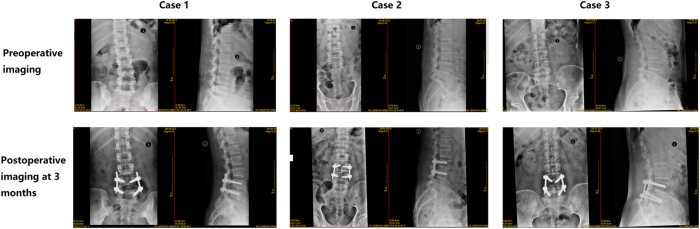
Representative anteroposterior and lateral lumbar spine X-ray images obtained preoperatively and at 3 months postoperatively in three patients.
